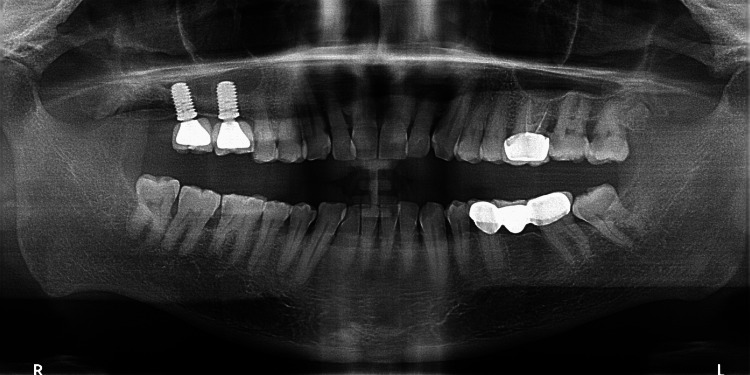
Two-year postoperative orthopantomogram.
